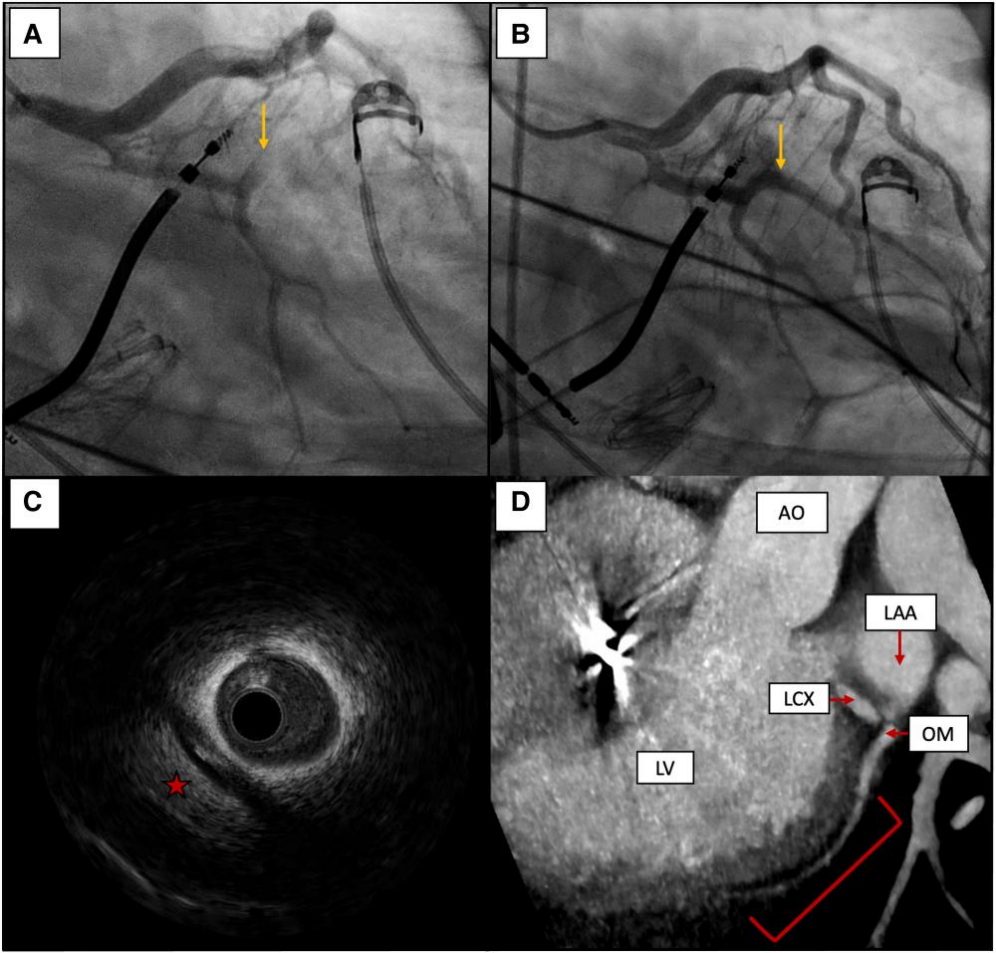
Multimodal imaging of acute coronary occlusion during epicardial ventricular tachycardia ablation. (*A*) Coronary angiography showing occlusion of the OM branch (yellow arrow). (*B*) Residual mild stenosis of the first OM branch after POBA with restored TIMI flow grade 3 (yellow arrow). (*C*) IVUS evidence of iso-hyperechoic extravascular image (red star) with extrinsic compression effect on the vessel. (*D*) CT-scan imaging showing an extended haematoma in the lateral wall of the left ventricle (red parenthesis), compressing the OM.
